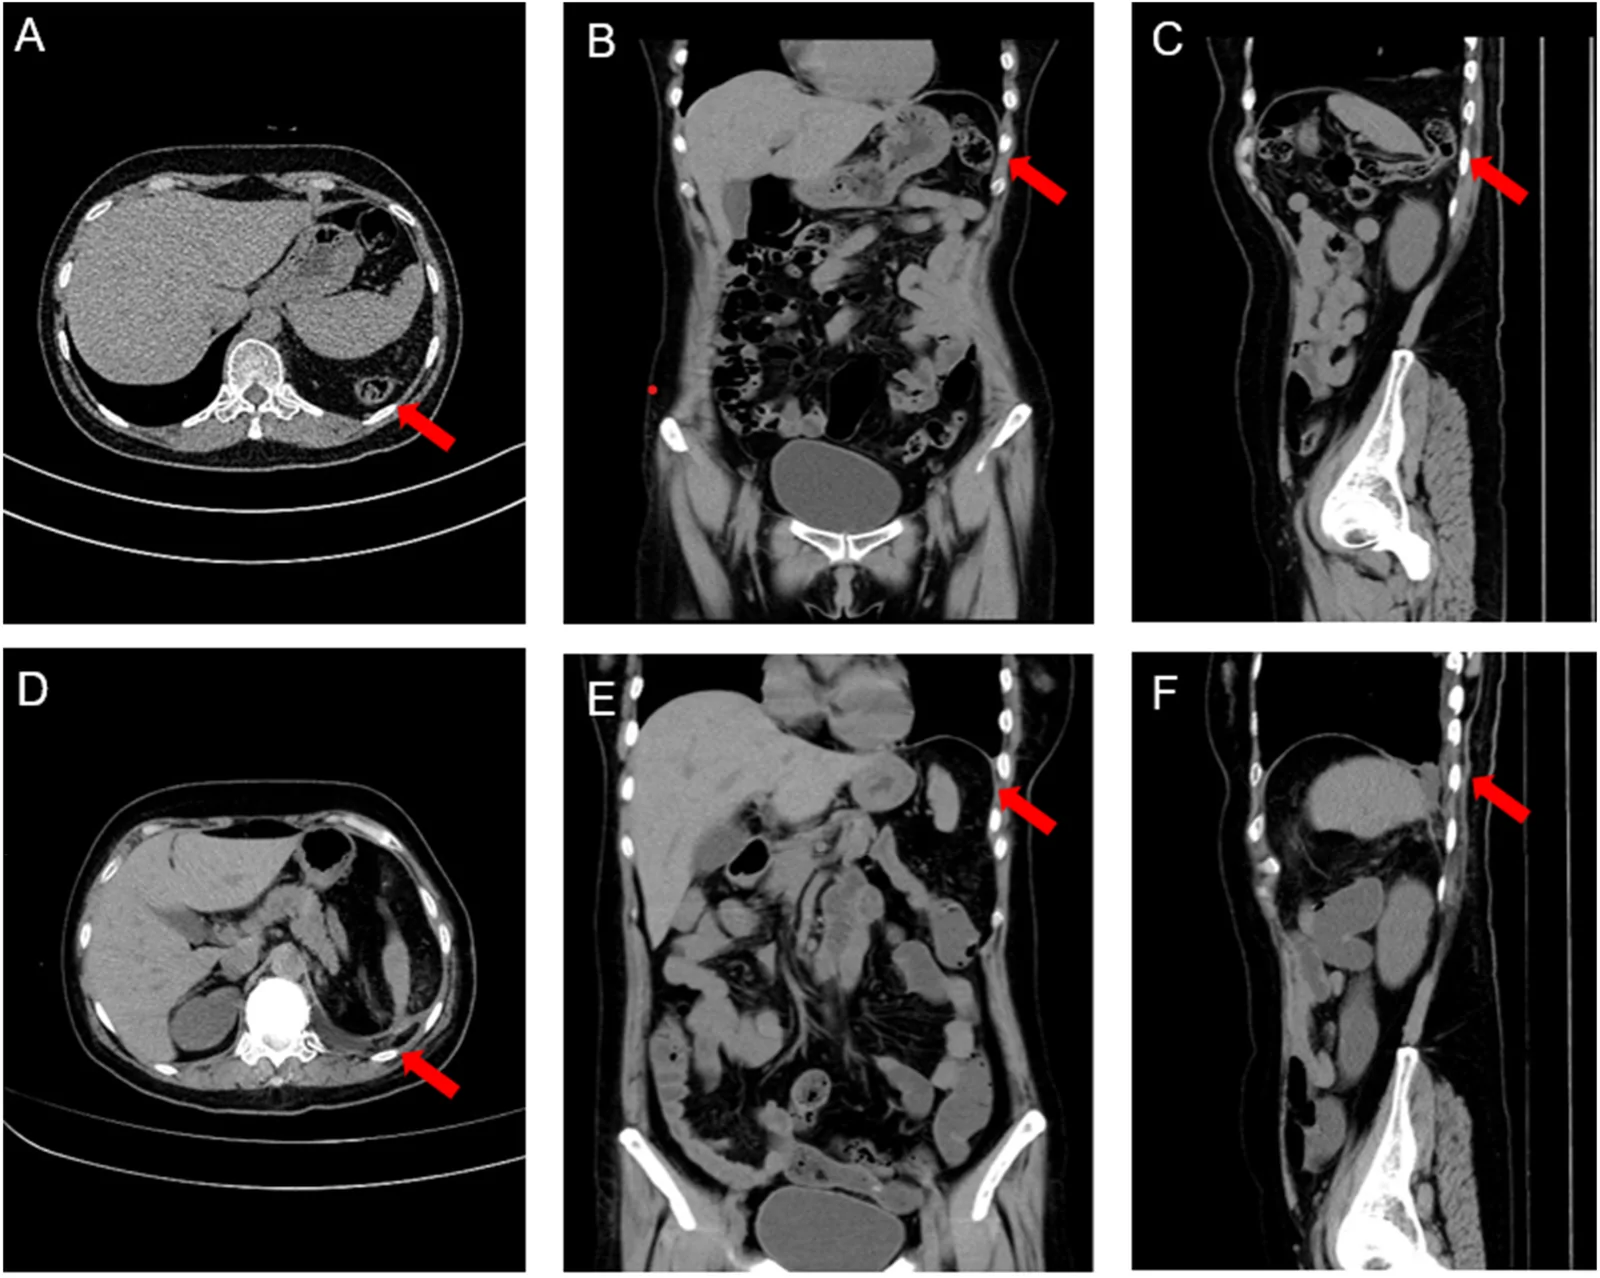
Preoperative and postoperative chest CT images. (A–C) Preoperative sagittal, coronal, and axial views demonstrating left diaphragmatic hernia with herniation of the colon and greater omentum. (D–F) Postoperative sagittal, coronal, and axial views showing complete repair of the left diaphragmatic defect.
